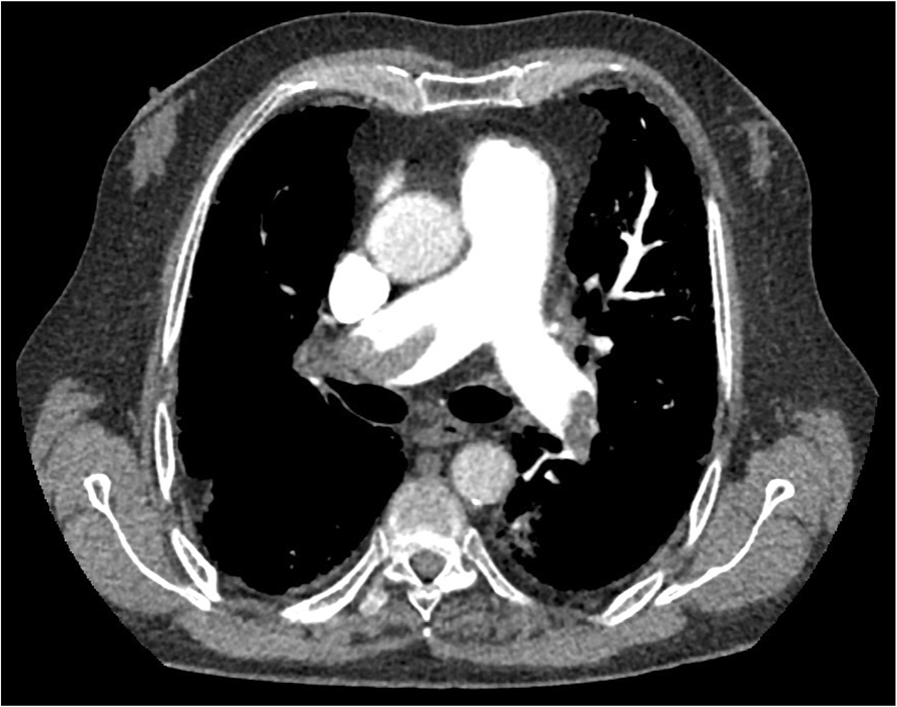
Computed tomography showing the saddle pulmonary embolus
